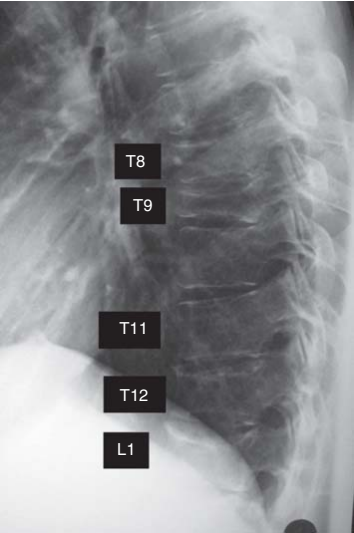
Lateral radiograph of the vertebrae in case 2. Radiograph showed mild compression changes at the 8th, 9th, and 12th thoracic vertebrae and 1st lumbar vertebra.
